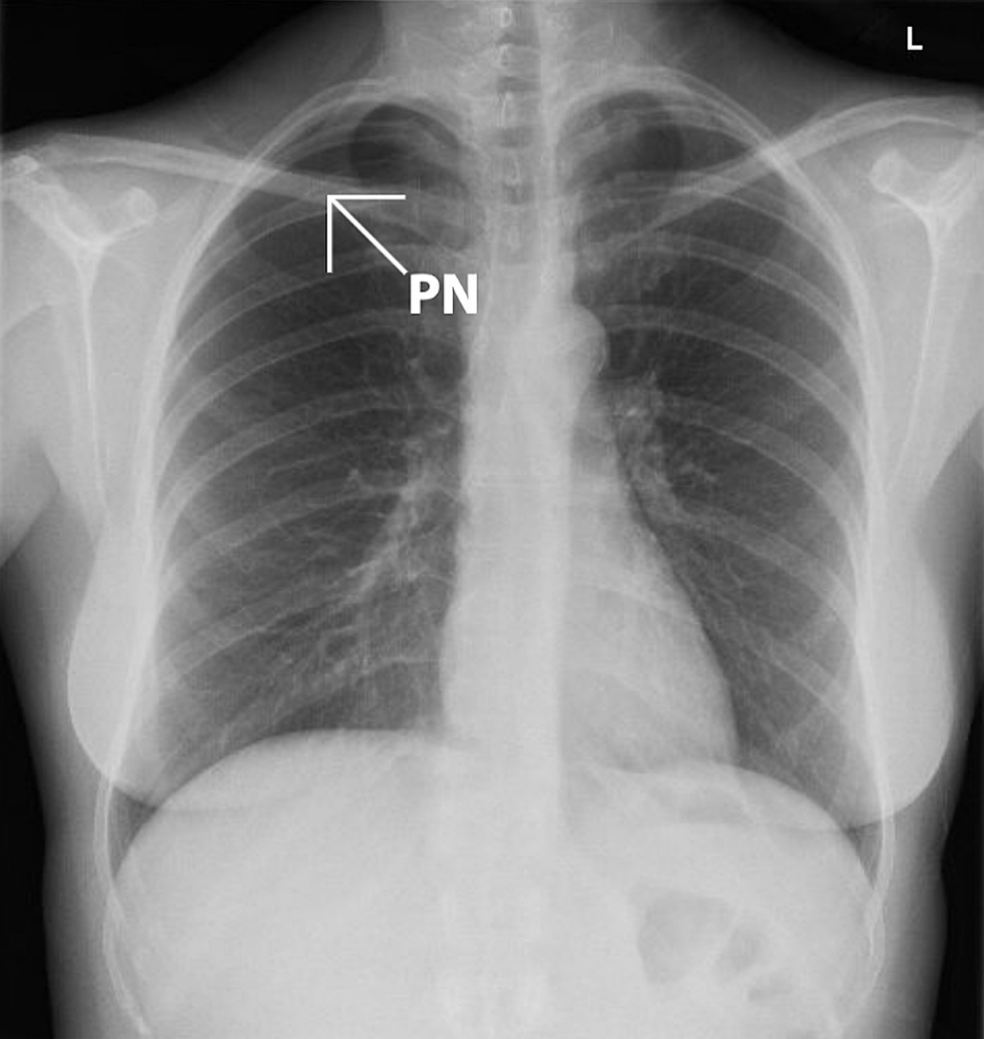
Posteroanterior (PA) chest X-ray demonstrating the presence of a right-sided pneumothorax (PN)
